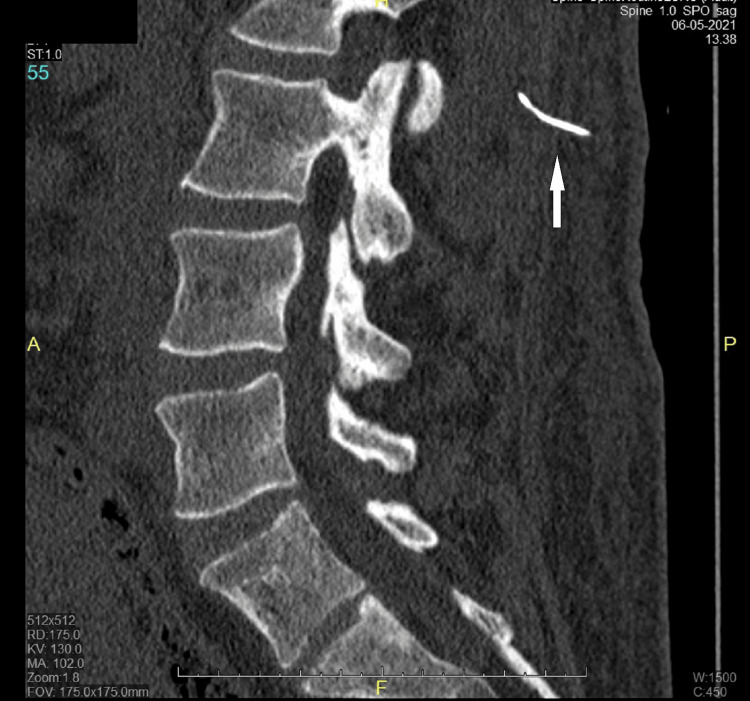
CT scan of the lumbar spine - image 1 The sagittal plane image shows a broken needle fragment (white arrow pointing to the needle fragment) at the L2 level, at a depth of 13 mm from the skin CT: computed tomography
